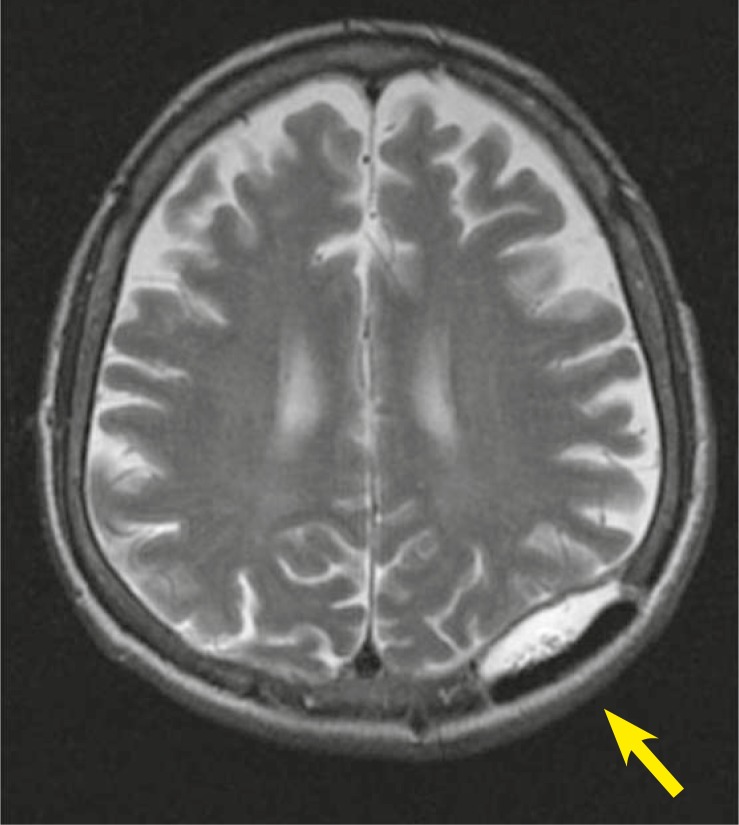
Brain MRI after second surgery which is contrast enhanced residual lesion.
